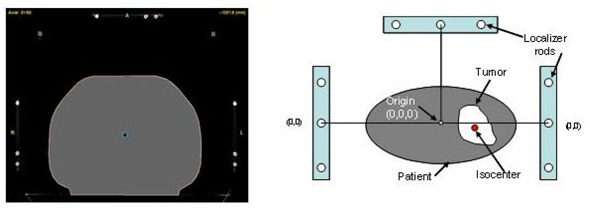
The body localizer consisting of nine rods shown on one slice of the CT image and the coordinate system established by the rods.
